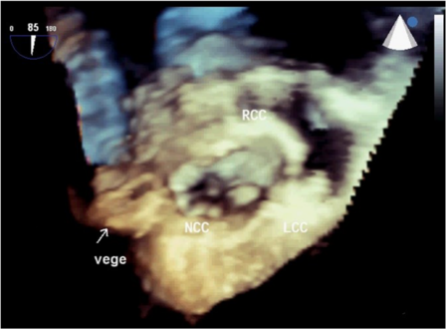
Three-dimensional imaging structured with transesophageal echocardiography. This revealed the exact location of the vegetation attached to the aortic root structures
